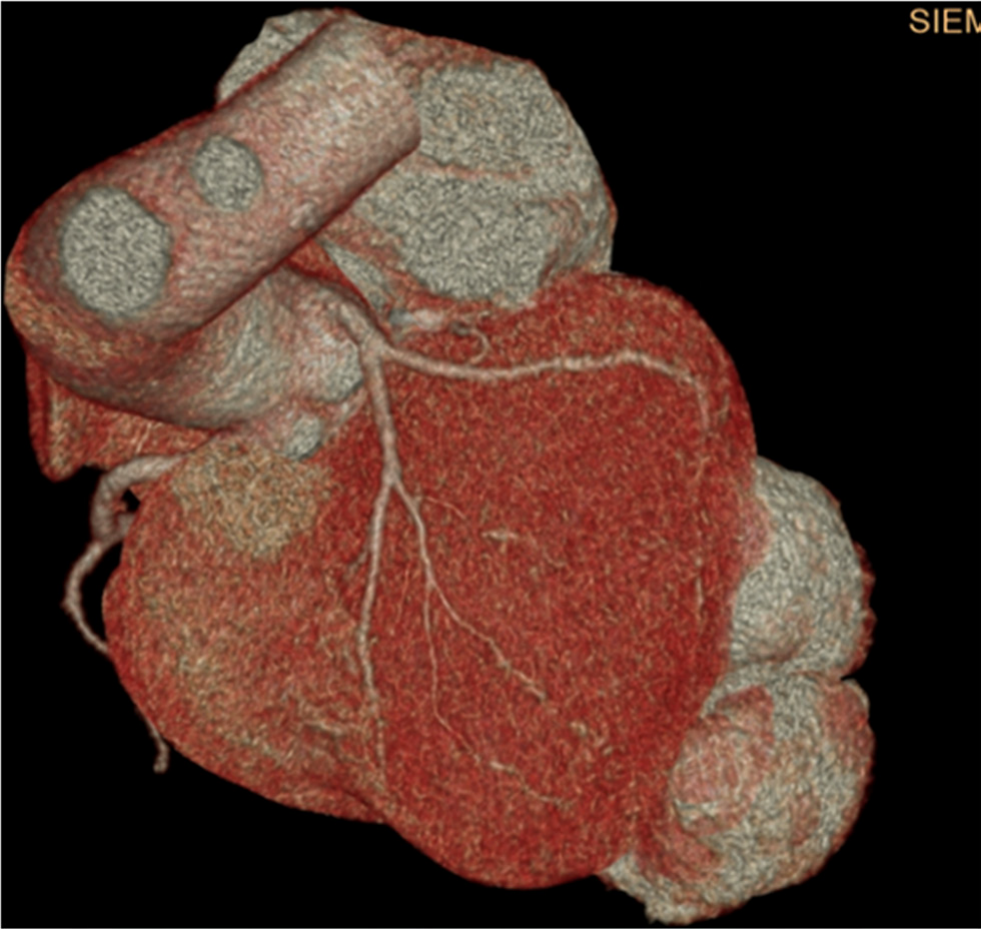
3-dimensional reconstructed computed tomography showing a large bilobed posterolateral left ventricular pseudoaneurysm.
